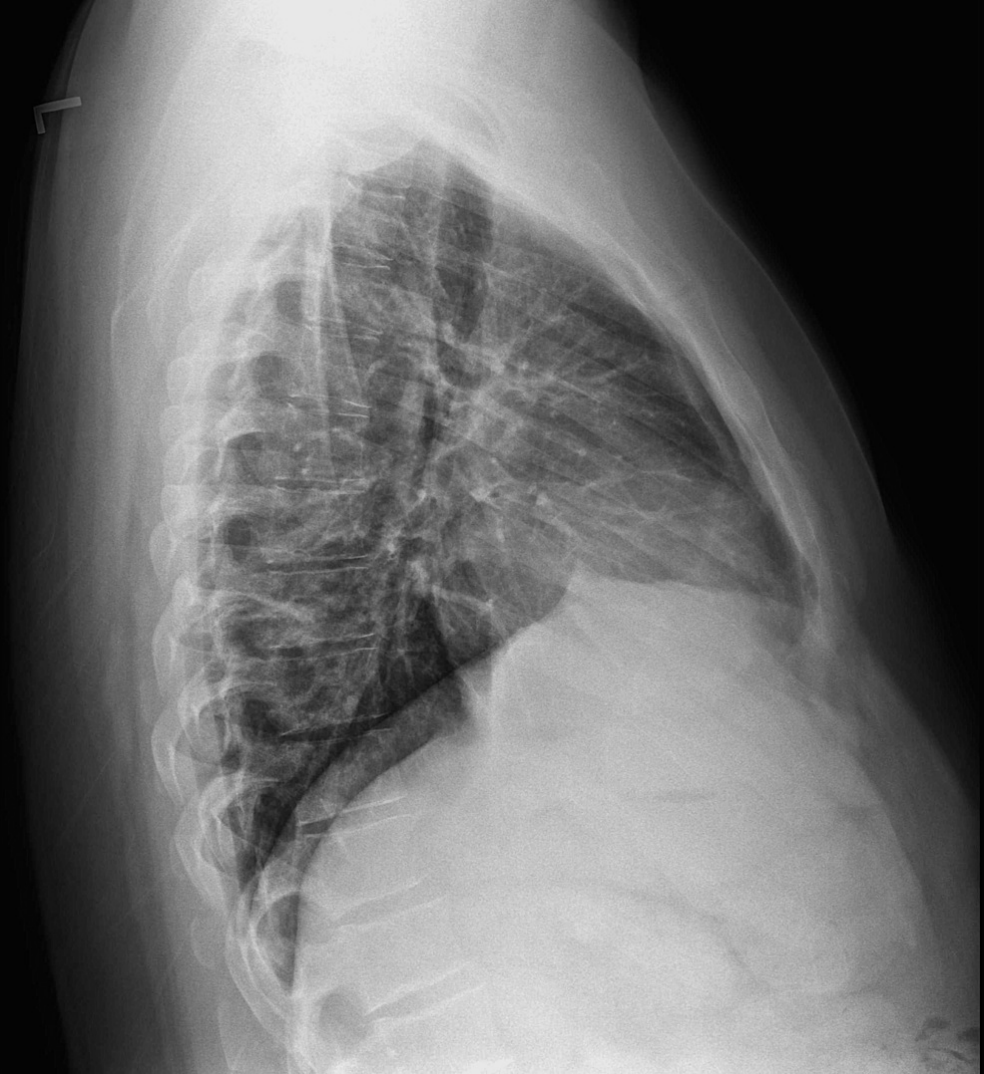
Lateral chest X-ray showing improvement of lower lobe consolidation at a follow-up visit nine days later.
